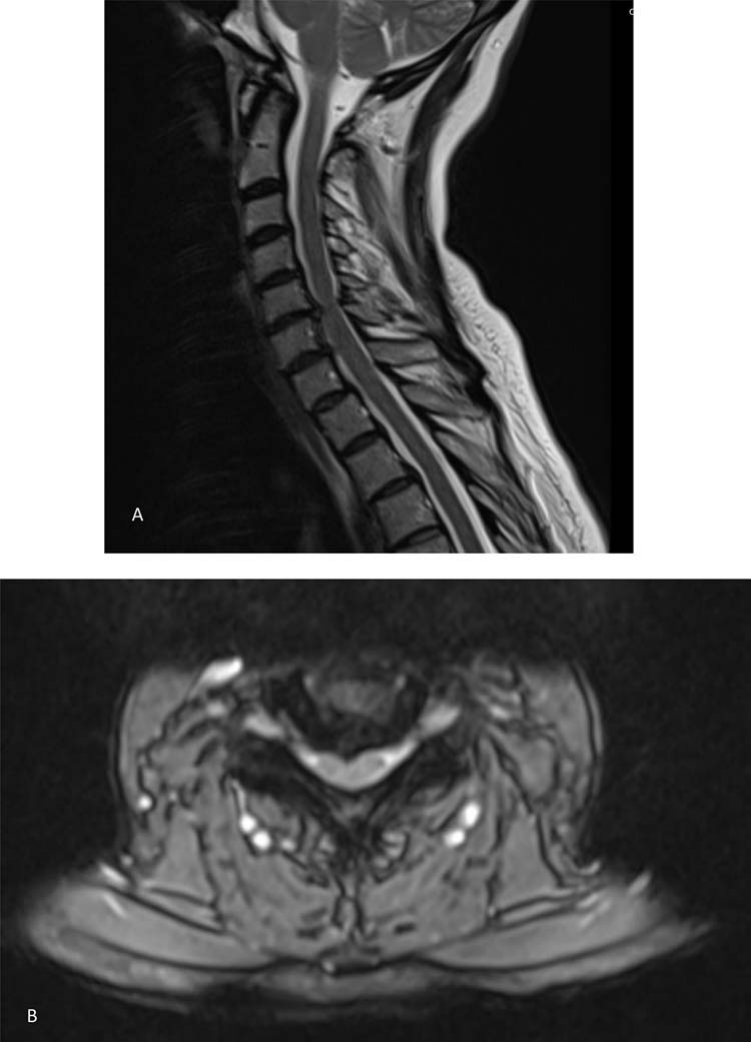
MRI showing bilateral C6 nerve root compression and left C7 nerve compression, C5–C8 disc herniation indenting the ventral aspect of the cord without typical signs of compression myelopathy or significant intramedullary signal abnormality. A. Sagittal view. B. Axial view.
